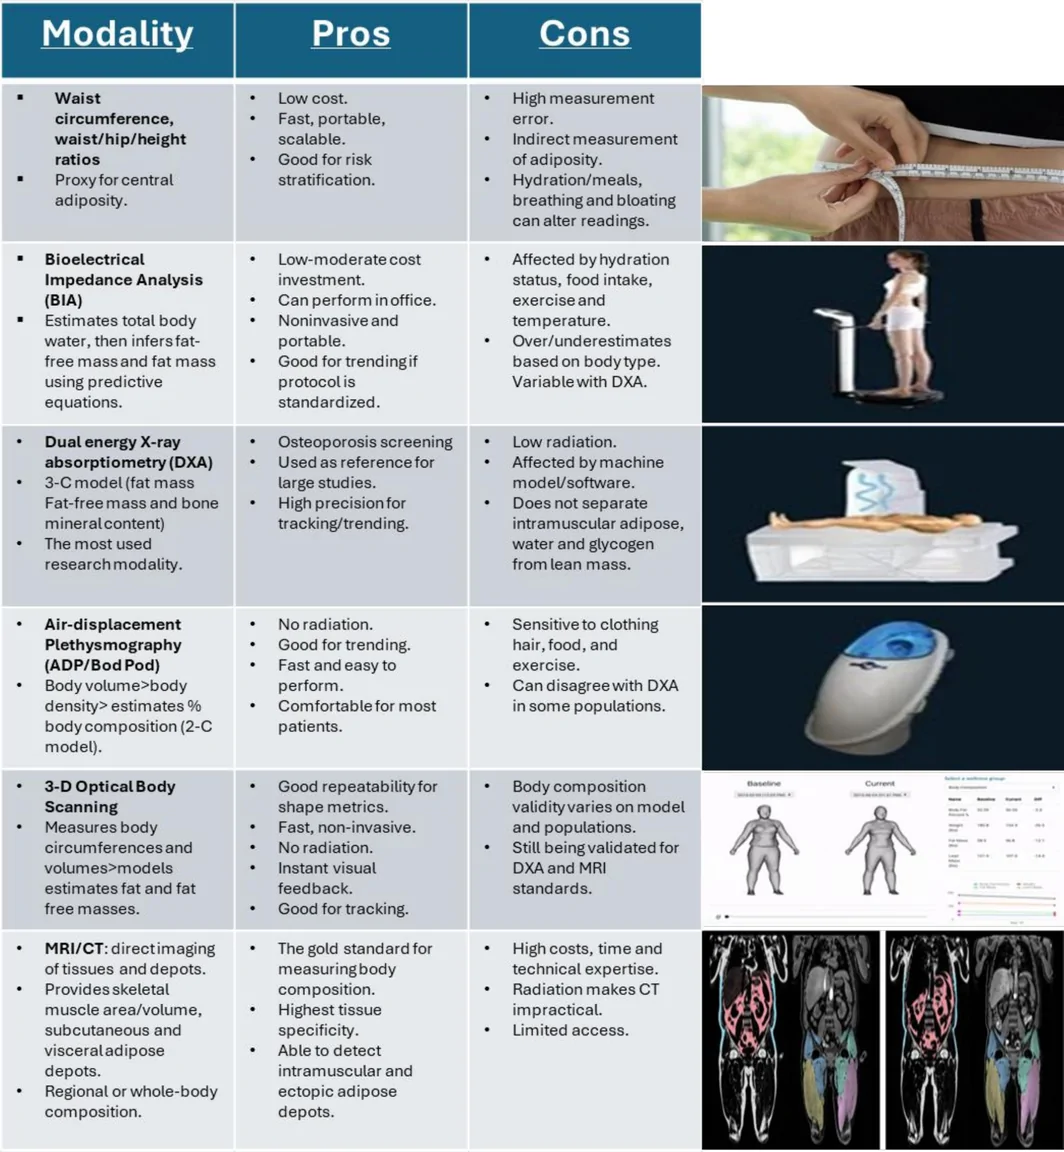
Body composition measuring modalities, including waist/hip/height circumferences and ratios, bioelectrical impedance analysis (BIA), dual‐energy x‐ray absorptiometry (DXA), air‐displacement plethysmography (ADP/Bod‐Pod), 3D body composition, magnetic resonance imaging (MRI), and computed tomography (CT).
